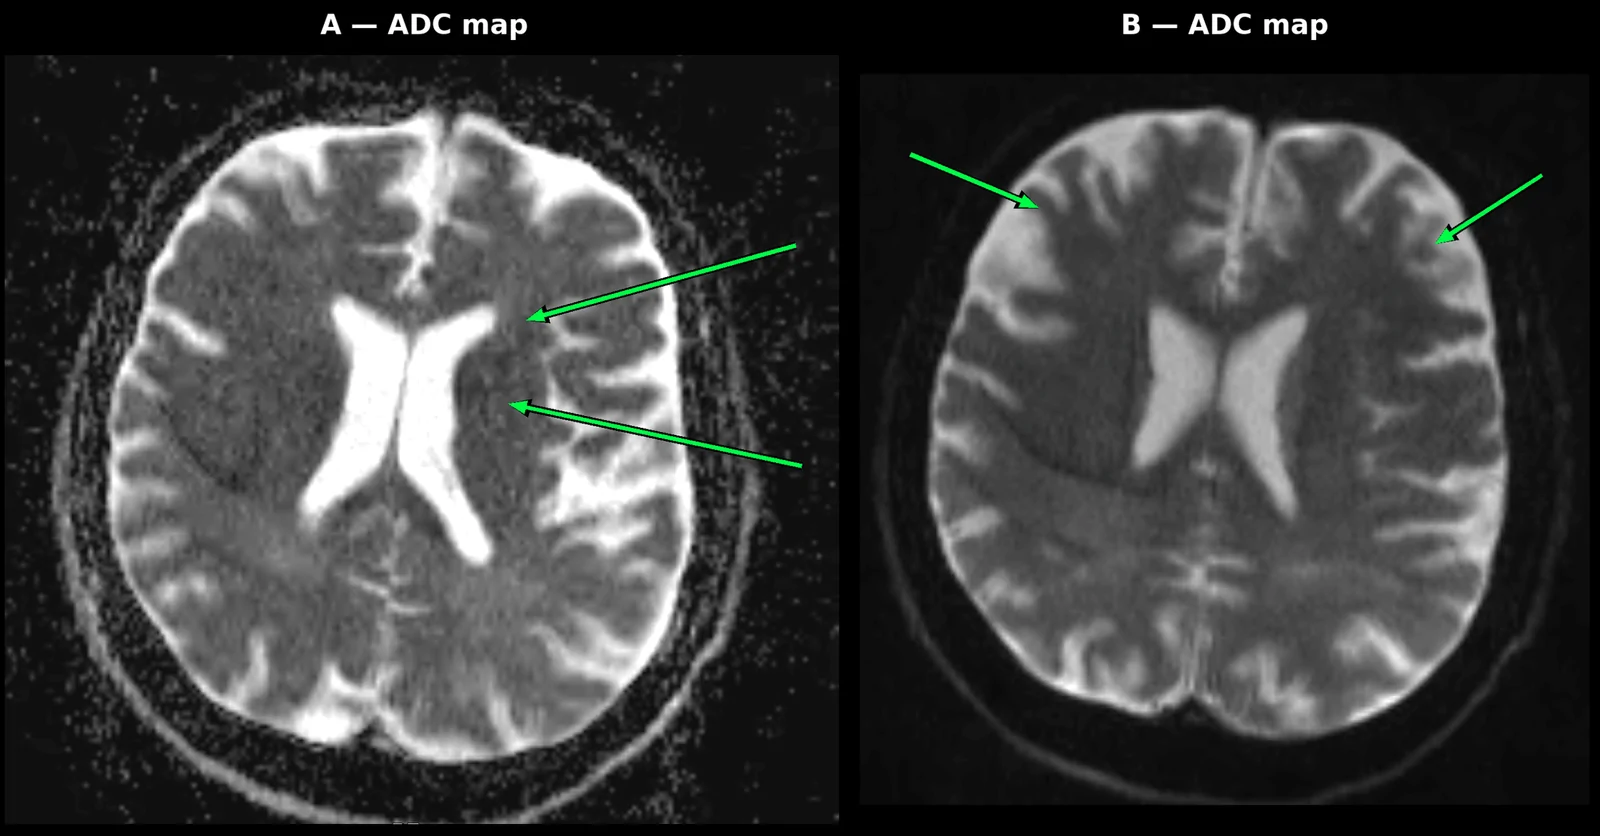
Axial diffusion MRI images (ADC maps) with green arrows indicating the described abnormalities. (A) Reduced signal in the head and body of the left caudate nucleus, consistent with restricted diffusion, with mildly increased volume compared with the contralateral caudate nucleus. (B) Moderate dilatation of the convexity subarachnoid spaces. ADC: Apparent diffusion coefficient.
